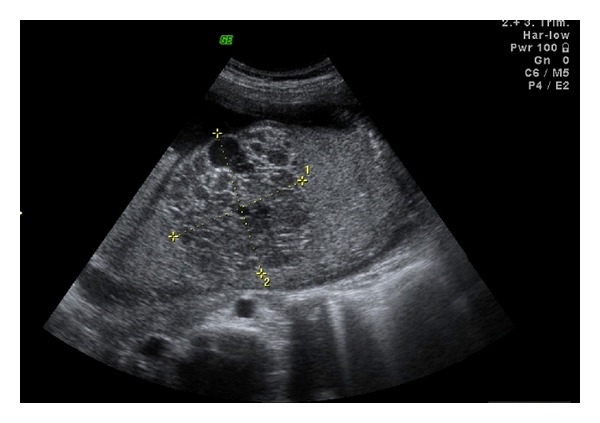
The gray-scale ultrasonography of Case 1 showing 7 × 7 × 6 cm hypoechoic, well-circumscribed intraplacental mass with some anechoic areas.
